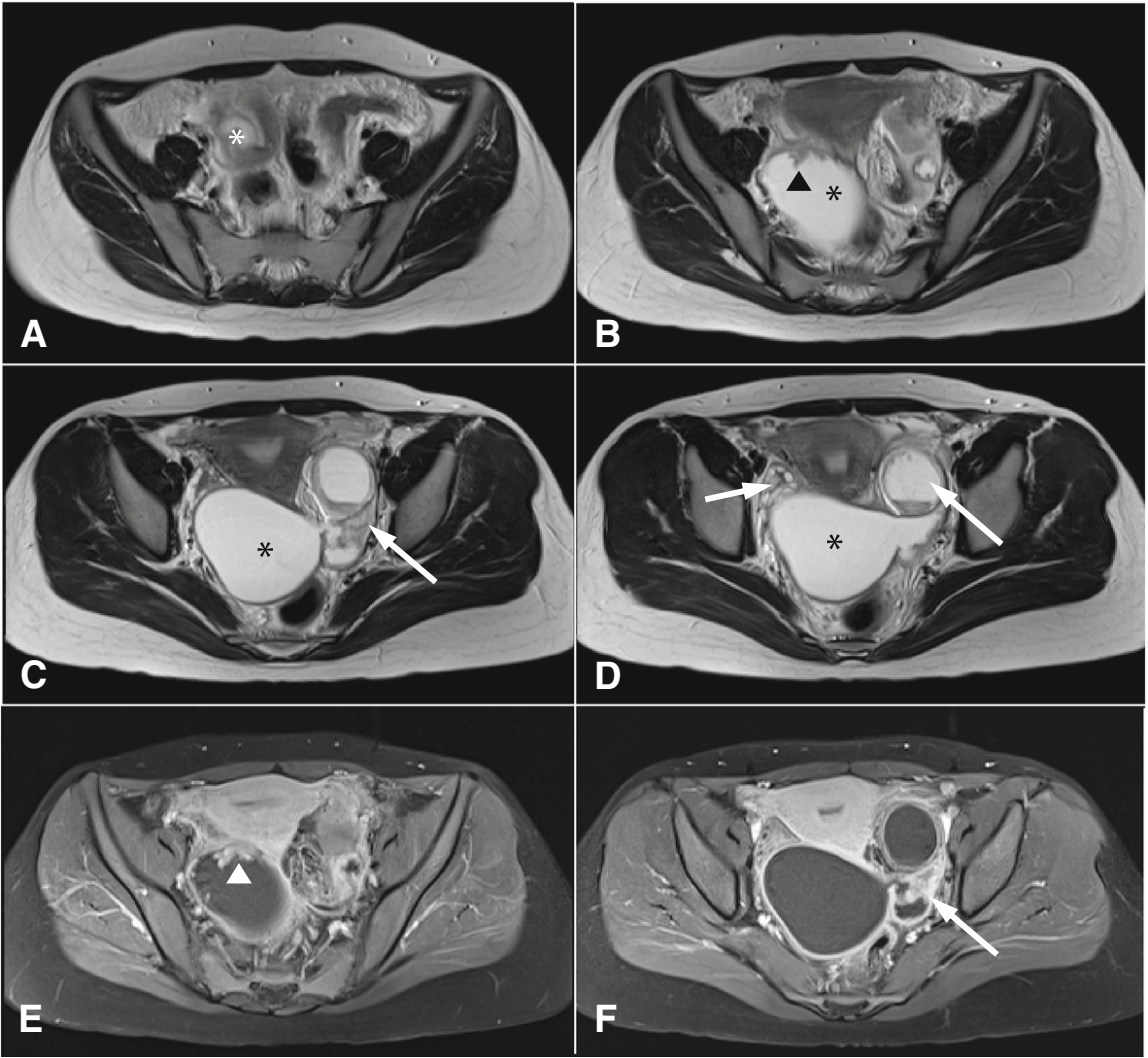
a-d Axial T2-weighted images showing bilateral adnexal complex cystic masses with fusiform or sausage-like shapes. The right adnexal mass (*) appeared as a cystic mass with papillary projections (*arrowhead*), whereas the left adnexal mass (long arrow) had an irregular thick wall. The right ovary (*short arrow*) was normal, but the normal left ovary was not visualized by MRI. (e-f) Contrast-enhanced fat-suppressed T1-weighted imaging revealed papillary projection enhancement (arrowhead) in the right adnexal mass and enhancement of the irregular thick wall (*long arrow*) in the left adnexal mass. These MRI features were suggestive of fallopian tube cancer
